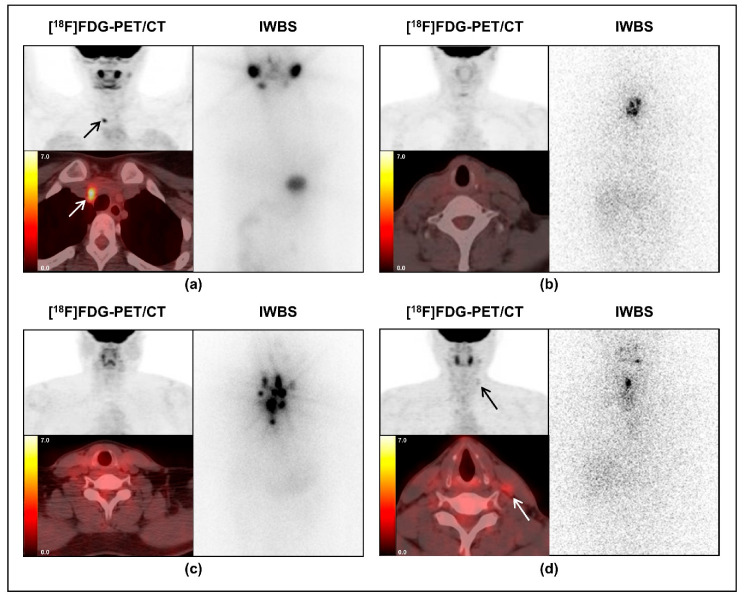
Differences in the diagnostic performance of PET/CT for detecting FDG-avid persistent disease (PD) based on the metastatic lymph node ratio (LNR)-combined criteria, and comparison with post-therapeutic iodine-whole-body scan (IWBS) in patients with papillary thyroid cancer (PTC). (a) An 18-year-old woman with a high central LNR (CLNR) of 1.00 was indicated for [^18^F]FDG-PET/CT because the serum thyroglobulin level immediately before RAI therapy (D0Tg, 16.0 ng/mL) was above the cut-off value (≥7.5 ng/mL). This patient had focal FDG uptake at the right neck level VI (black and white arrows, SUVmax: 4.9), which was pathologically revealed as PD. There was no significant iodine uptake in the thyroidectomy bed or in the midline neck area on IWBS. (b) A 46-year-old woman with a low CLNR of 0.16, which was less than the cut-off value (0.44), was not indicated for [^18^F]FDG-PET/CT despite of a high D0Tg level (10.0 ng/mL). This patient had no FDG-avid PD on PET/CT; however, several iodine uptake areas were observed in the anterior neck on IWBS. (c) A 40-year-old man with a high CLNR of 1.00 was initially indicated for [^18^F]FDG-PET/CT because of a high serum D0Tg level (21.0 ng/mL) according to the criteria. However, there was no FDG-avid PD on PET/CT. Instead, there were multiple iodine uptake areas in the anterior neck on IWBS. (d) A 29-year-old man with a high CLNR of 0.53 had FDG-avid PD at the left neck level III (black and white arrows, SUVmax: 2.9), although he was not indicated for [^18^F]FDG-PET/CT because of a low D0Tg level (1.0 ng/mL). An IWBS showed iodine uptake only in the midline of the anterior neck. (IWBS, iodine-whole-body scan).
